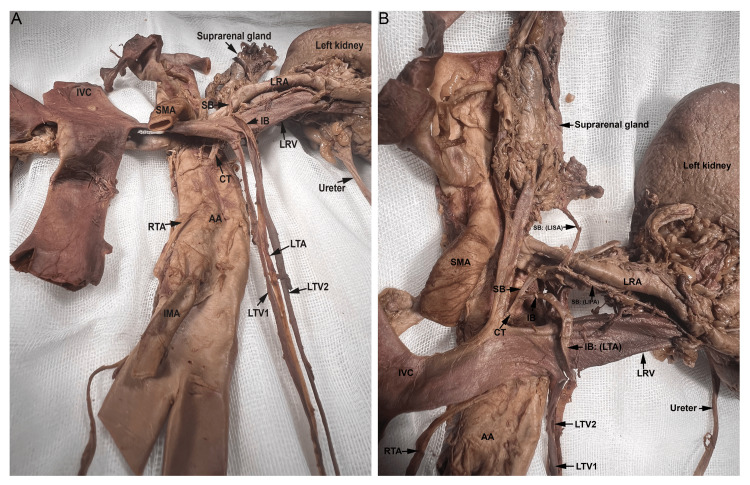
Arched left testicular artery. (A) The common trunk (CT) shown from the inferior view with the left renal vein (LRV) lifted. The CT gave rise to the left testicular artery (LTA), left inferior suprarenal artery (LISA), and left inferior polar renal artery (LIPA) and was covered by the LRV. (B) Photographs taken during dissection show that the CT gave rise to two branches, superior branch (SB) and inferior branch (IB). The SB further bifurcated into the LISA and the LIPA. The IB arched over the LRV and continued as the LTA. AA: Abdominal Aorta; IMA: Inferior Mesenteric Artery; IVC: Inferior Vena Cava; LRA: Left Renal Artery; LTV 1: Left Testicular Vein 1; LTV 2: Left Testicular Vein 2; RTA: Right Testicular Artery; SMA: Superior Mesenteric Artery; IB: Inferior Branch.
